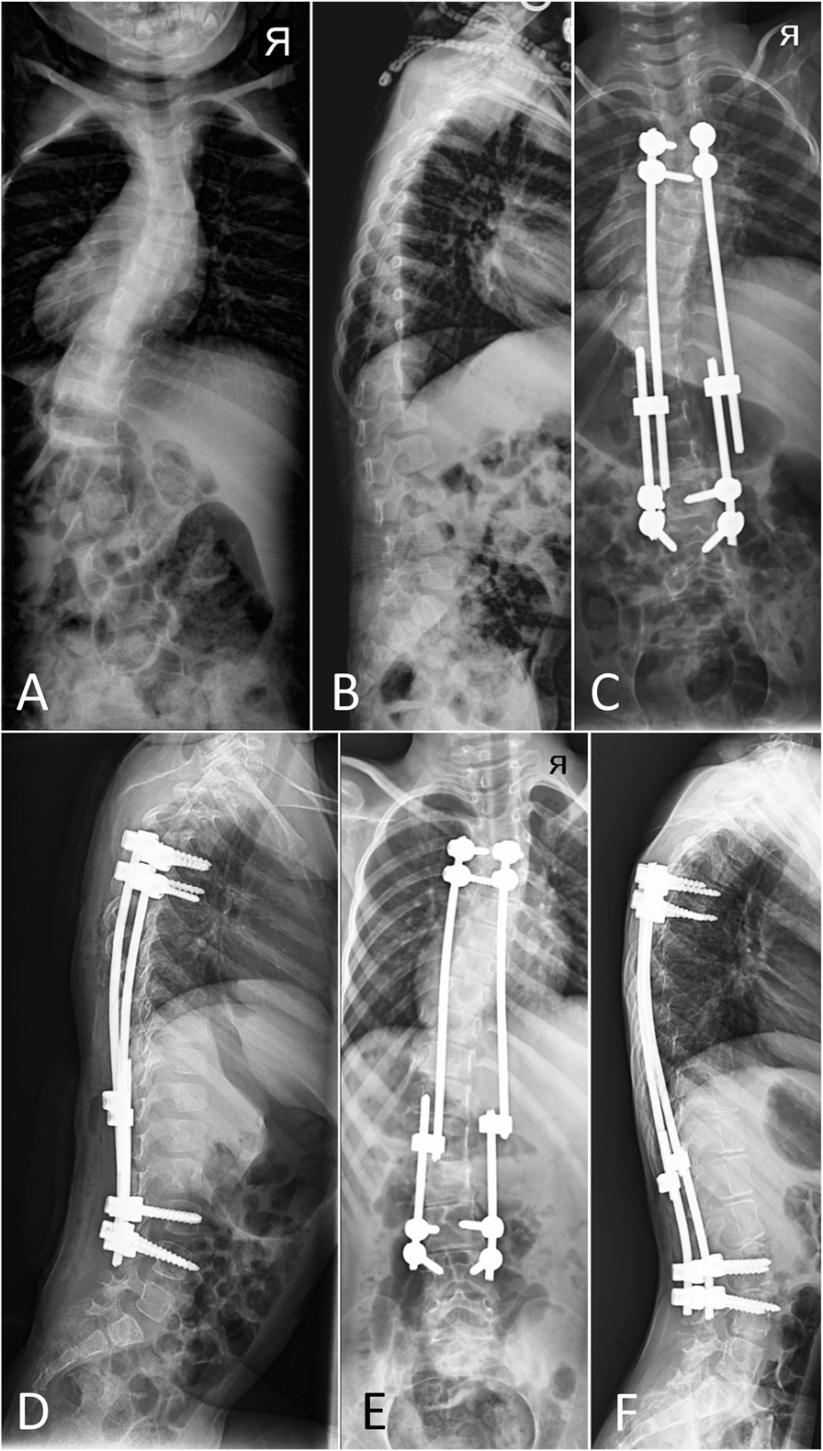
Proximal junctional kyphosis. Posteroanterior and lateral spine radiographs of a three-year-old boy with idiopathic early-onset scoliosis (a-b) who underwent dual growing rod instrumentation with all-pedicle screws (c-d). Three-year follow-up radiographs (e-f) reveal a proximal junctional angle of  18°, consistent with a diagnosis of proximal junctional kyphosis. The patient was asymptomatic and did not undergo revision
